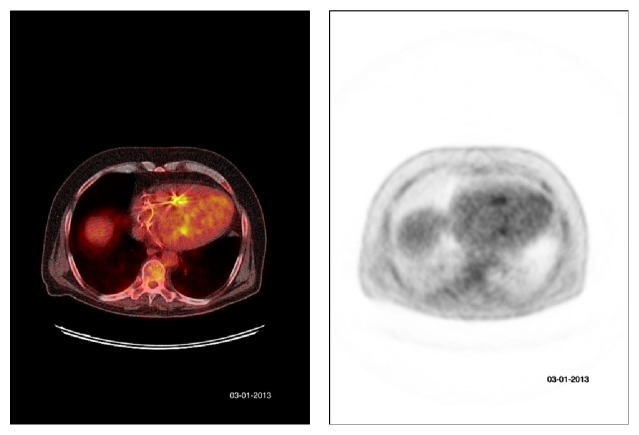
^18^F-FDG-PET scanning demonstrates pathologic activity by the tip of both ICD-leads.
